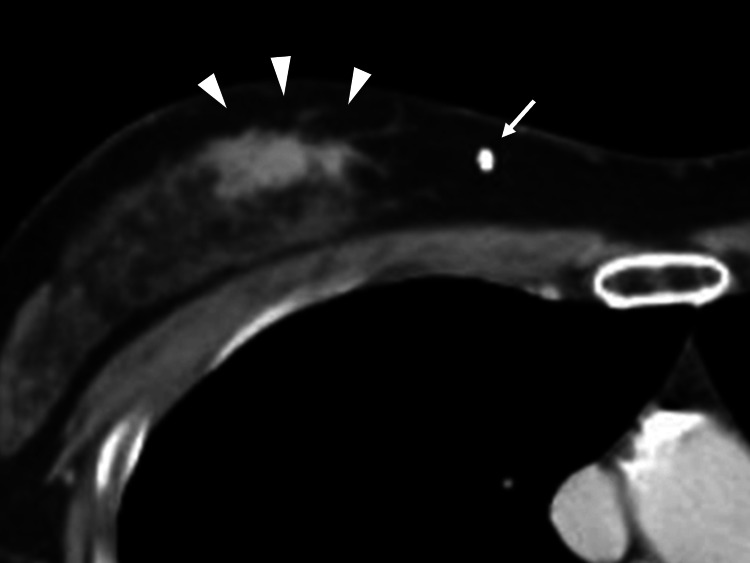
Contrast-enhanced computed tomography (axial section): preoperative tumor-catheter relationship An irregularly shaped, enhancing tumor is seen in the right breast (arrowheads). The shunt catheter is identified as a high-density structure on the medial side of the tumor (arrow).
